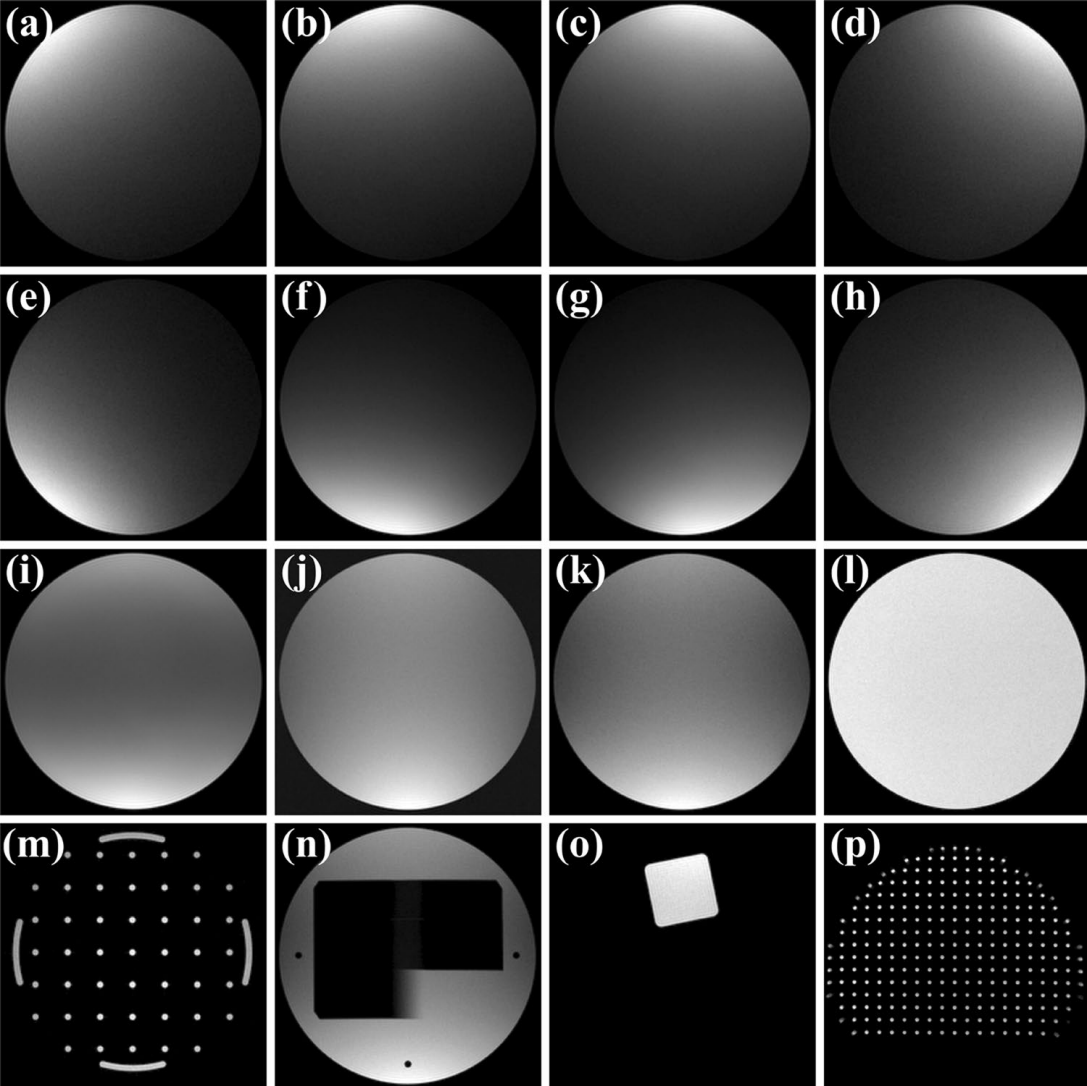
Representative MR images from quality tests. a–i Flood field uniformity (FFU) test images from the MRL_QA_SNR scans: a–d anterior coil elements (T_1–T_4), e–h posterior coil elements (T_5–T_8), and i combined result across all elements. j–l Uniformity test images from the PIQT scans: j QA1, Spin-echo (TE = 100 ms) using anterior and posterior coils; k QA2, Gradient-echo (TE = 15 ms) using anterior and posterior coils; l QA3, Spin-echo (TE = 100 ms) using the system body coil. m Spatial linearity test image from the MRL_QA_SCALING scan. n Slice profile test image from the PIQT scan. o Spatial resolution test image from the PIQT scan. p 3D geometric distortion test image
